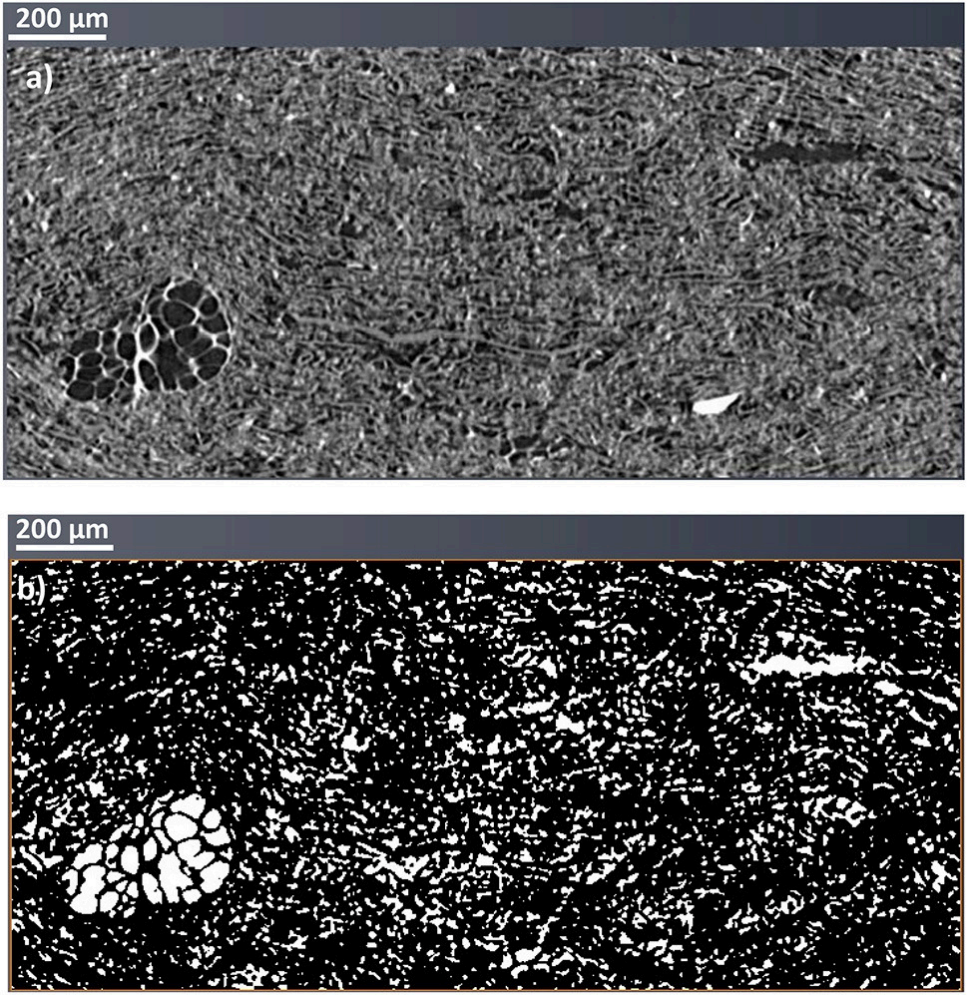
3D X-ray reconstituted images of 400 gsm sample (400 gsm-1x). (a) Grayscale and (b) Thresholded cross section images.
